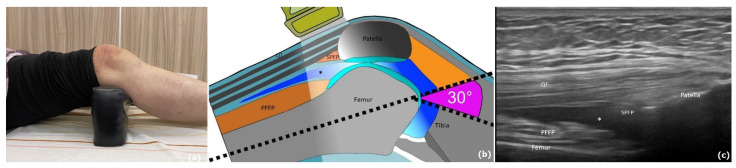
Ultrasound evaluation of the knee in a patient with stage II knee osteoarthritis and synovitis. (a) The patient lay supine with 30° knee flexion. (b) The ultrasound probe was placed over the knee just superior to the patella to examine the suprapatellar bursa. (c) Knee ultrasound longitudinal view indicating the position of the suprapatellar bursa between the patella and femur. PFFP, prefemoral fat pad; SPFP, suprapatellar fat pad; QT, trilaminar quadriceps tendon; Hypoechoic space (asterisk) reflects joint effusion.
